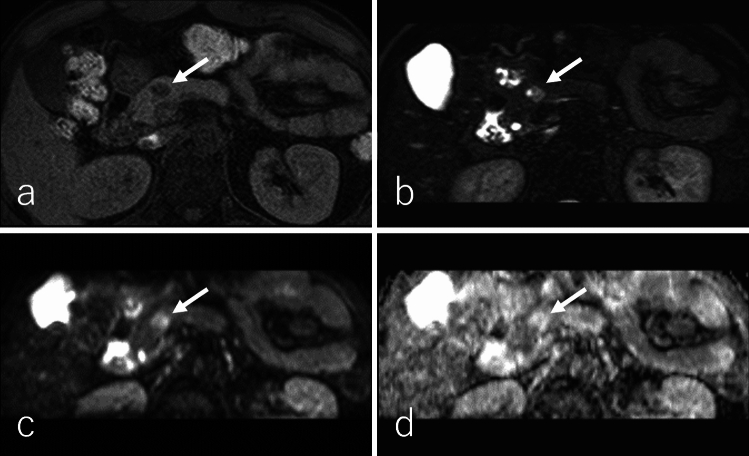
A 45-year-old male patient with solid-pseudopapillary neoplasm. a Fat-suppressed T1-weighted imaging (FS-T1WI), b fat-suppressed T2-weighted imaging (FS-T2WI), c diffusion-weighted imaging (DWI) with b value of 800 s/mm^2^, and d apparent diffusion coefficient (ADC) map. The tumor is located in the pancreatic head (arrow), measuring 1.5 cm in diameter, and exhibits expansile growth with well-defined margins. The tumor exhibits heterogeneous hypointensity on FS-T1WI, heterogeneous hyperintensity on FS-T2WI, and DWI, with higher ADC values (3.10 × 10⁻^3^ mm^2^/s), relative to the pancreatic parenchyma. Additionally, the tumor demonstrates a small cystic component on the right side of the tumor on FS-T2WI
